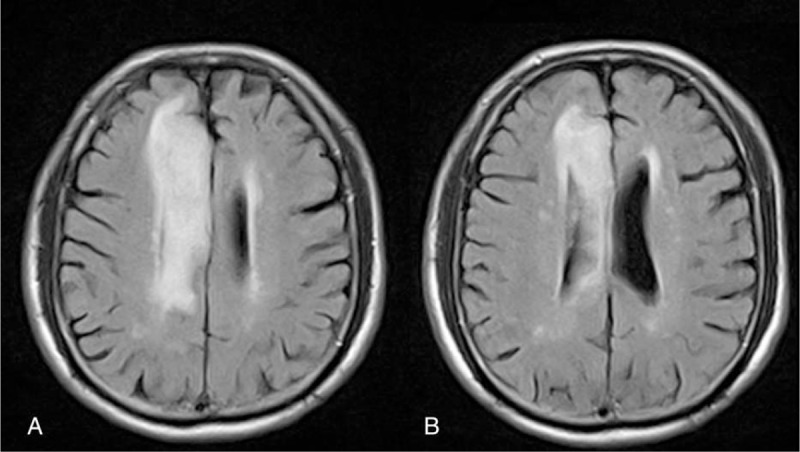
Brain MRI. (A and B) Serial FLAIR MRI shows the high signal intensity in the territory of the right anterior cerebral artery, which indicates recent cerebral infarction. MRI = magnetic resonance imaging.
